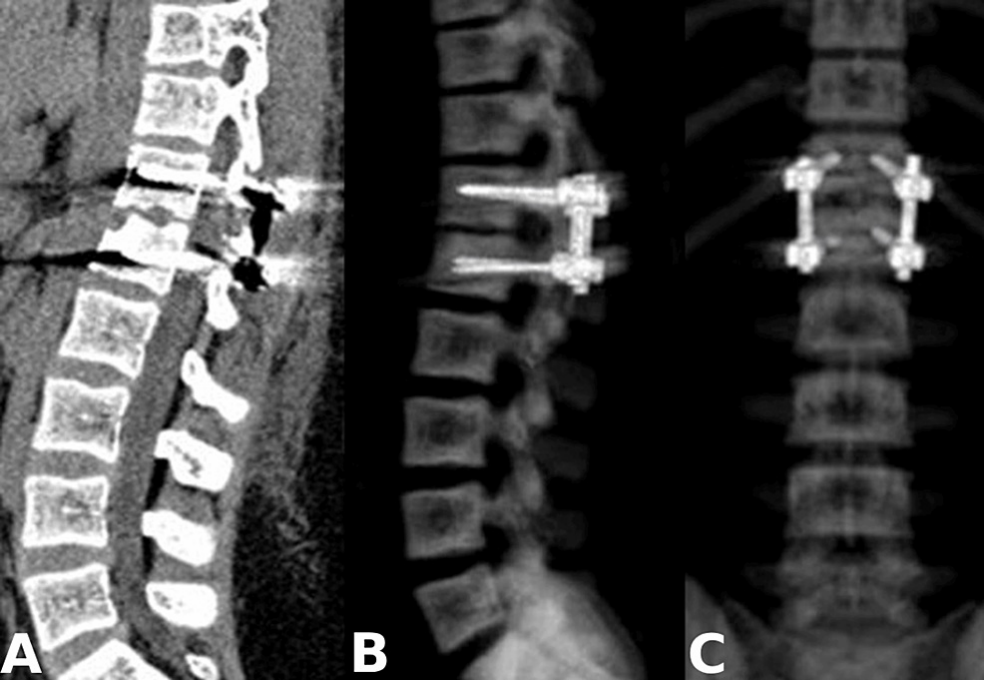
Postoperative CT. (А) Sagittal reconstruction and (B and C) sagittal and coronal 3D-reconstruction showing posterior transpedicular fixation of T12 and L1 vertebral bodies
